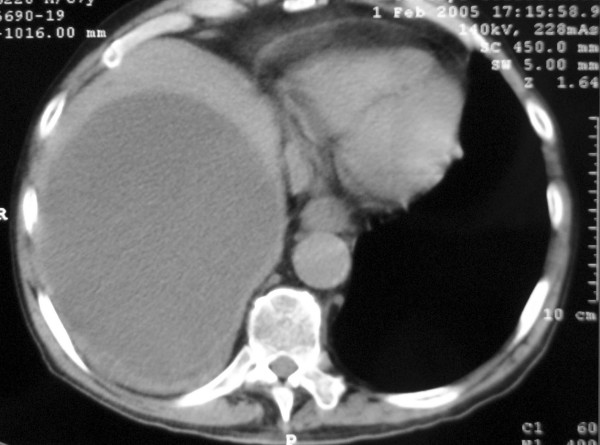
During second admission. Abdominal CT scan revealing the same clear right liver lobe cystic mass.
